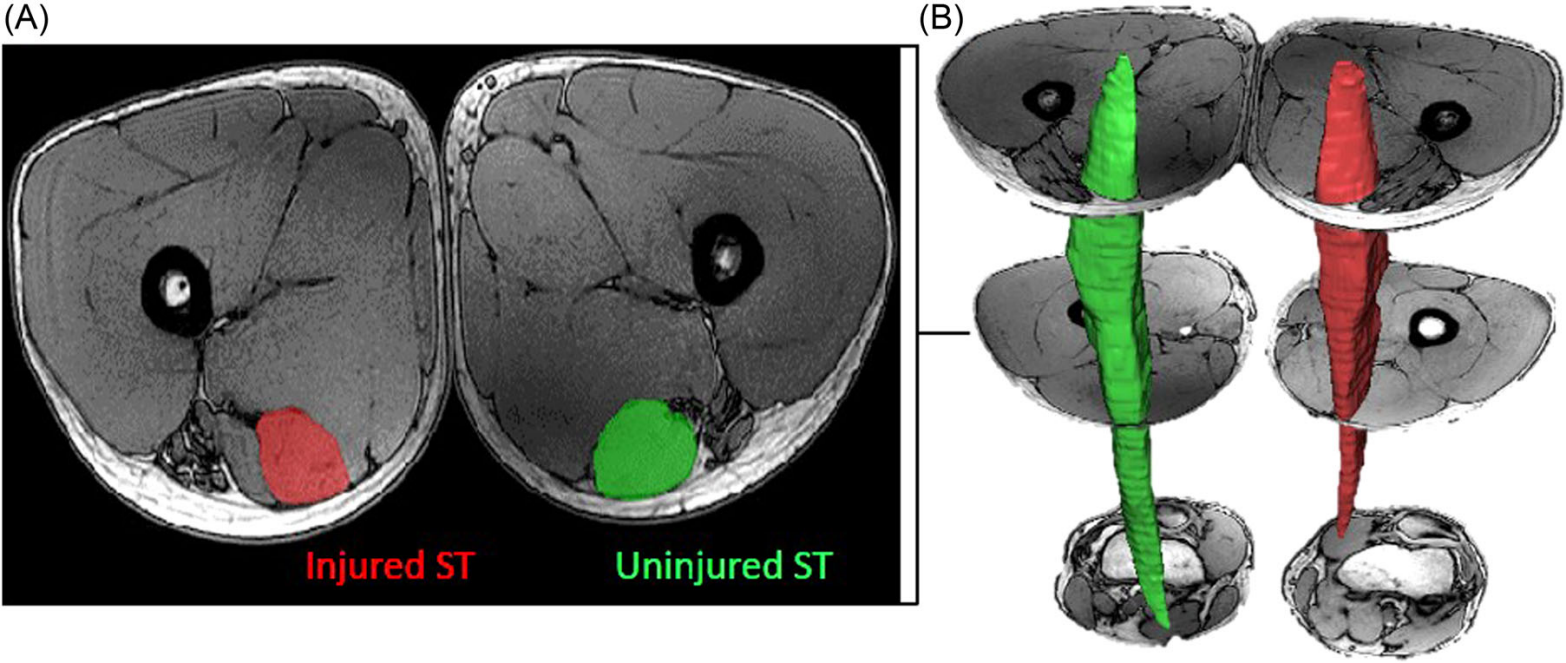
Muscle segmentation from a participant with unilateral anterior cruciate ligament reconstruction involving a semitendinosus (ST)‐gracilis (GR) autograft. (A) Cross‐sectional magnetic resonance image displaying the surgically harvested and uninjured contralateral ST. (B) The ST was segmented manually from magnetic resonance images to calculate muscle length, peak cross‐sectional area, and volume. In this image, 3 of 489 segmented slices are depicted. [Color figure can be viewed at wileyonlinelibrary.com]
